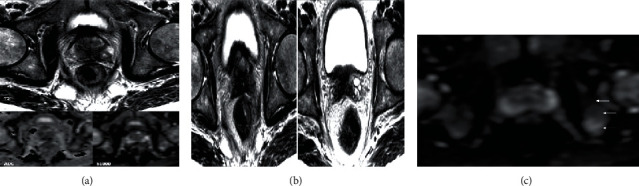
(a) Multiparametric MRI image showing a suspicious nodule with capsular intrusion and invasion of the rectal wall. Nodular image sitting at the level of the left CZ sector of the prostatic base in heterogeneous hyposignal on the T2-weighted images, in hypersignal B1000 diffusion with restriction on the ADC card. Note a rupture of the prostatic capsule with invasion of the left neurovascular strip and thickening of the rectal wall (isosignal T2 and hypersignal in diffusion). (b) T2 sequence of a multiparametric MRI of the prostate objectifying an invasion of the base of the seminal vesicles T2 hyposignal aspect of the base of the seminal vesicles denoting their invasion. (c) B1000 diffusion sequence of a multiparameter MRI objectifying an associated secondary bone involvement, with a heterogeneous aspect of the bone structure.
